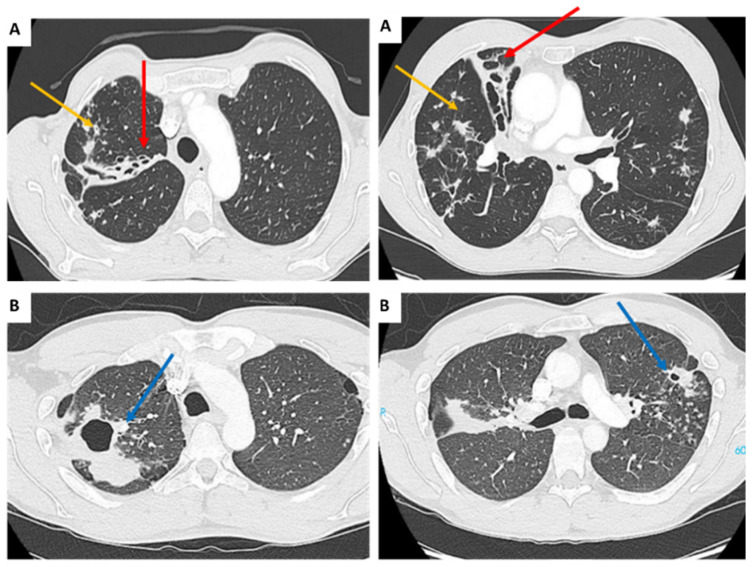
Computed tomography scan findings in two MAC-infected patients with (A) nodular bronchiectasis pattern with bronchial wall thickening (red arrows), centrilobular nodules and mucus plugging (yellow arrows) and (B) cavitary pattern with multiple cavitating lesions (blue arrow).
